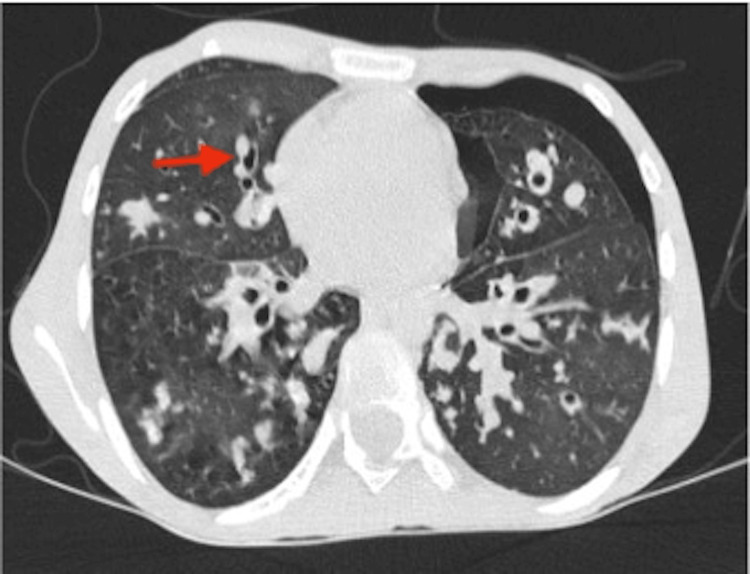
CT image showing signet ring sign. Note that the dilated airway diameter is greater than the diameter of the adjacent blood vessel Red arrow: signet ring sign
